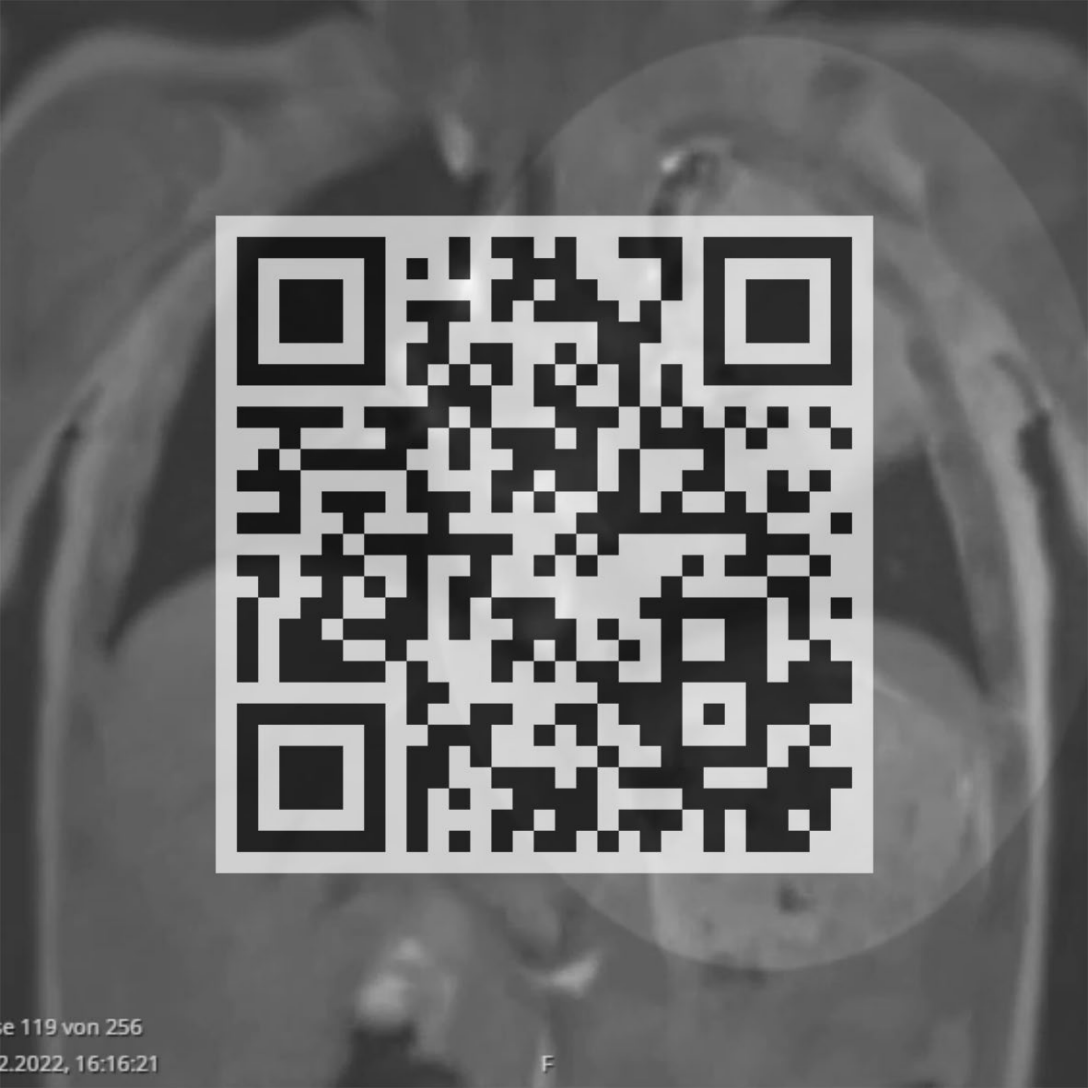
Video 8 T2 /T1 weighted volume coverage sequence in coronal orientation (video on the right side) in a 1.5 -year-old boy with a pneumonic consolidation in comparison with the x-ray (on the left side). There are no motion artifacts despite the free breathing of the patient. Hence the infiltration in the left upper lobe is well depicted. In contrast to the X-ray, a small abscess can be seen within the pneumonia by real-time MRI (bright signal, red arrow)
